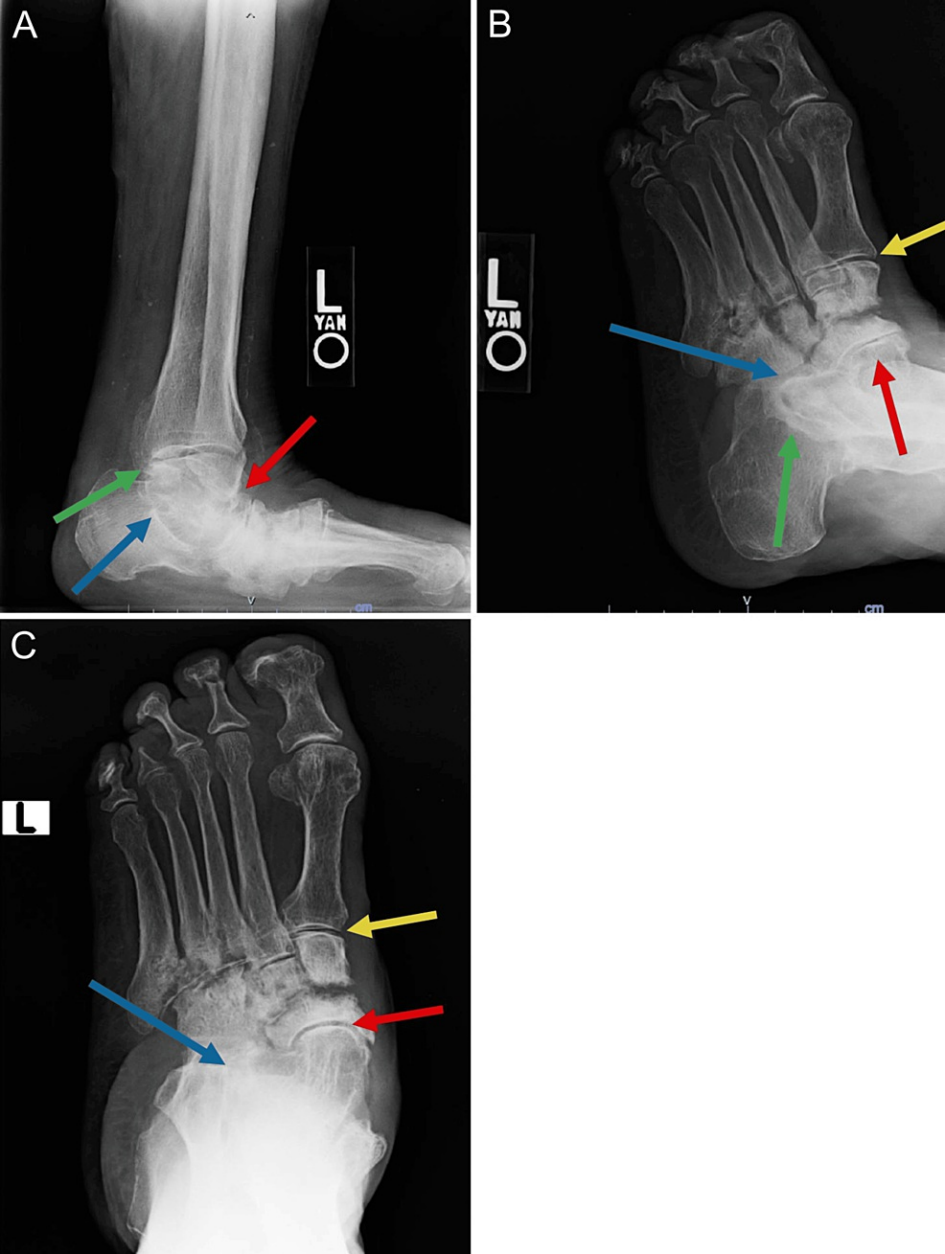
X-ray of the patient's left foot showing the (A) lateral view, (B) lateral superior view, and (C) superior view of Charcot neuroarthropathic osteolysis of the talonavicular (red arrow), talocalcaneal (green arrow), and calcaneocuboid (blue arrow) joints as well as the Lisfranc joint (yellow arrow)
